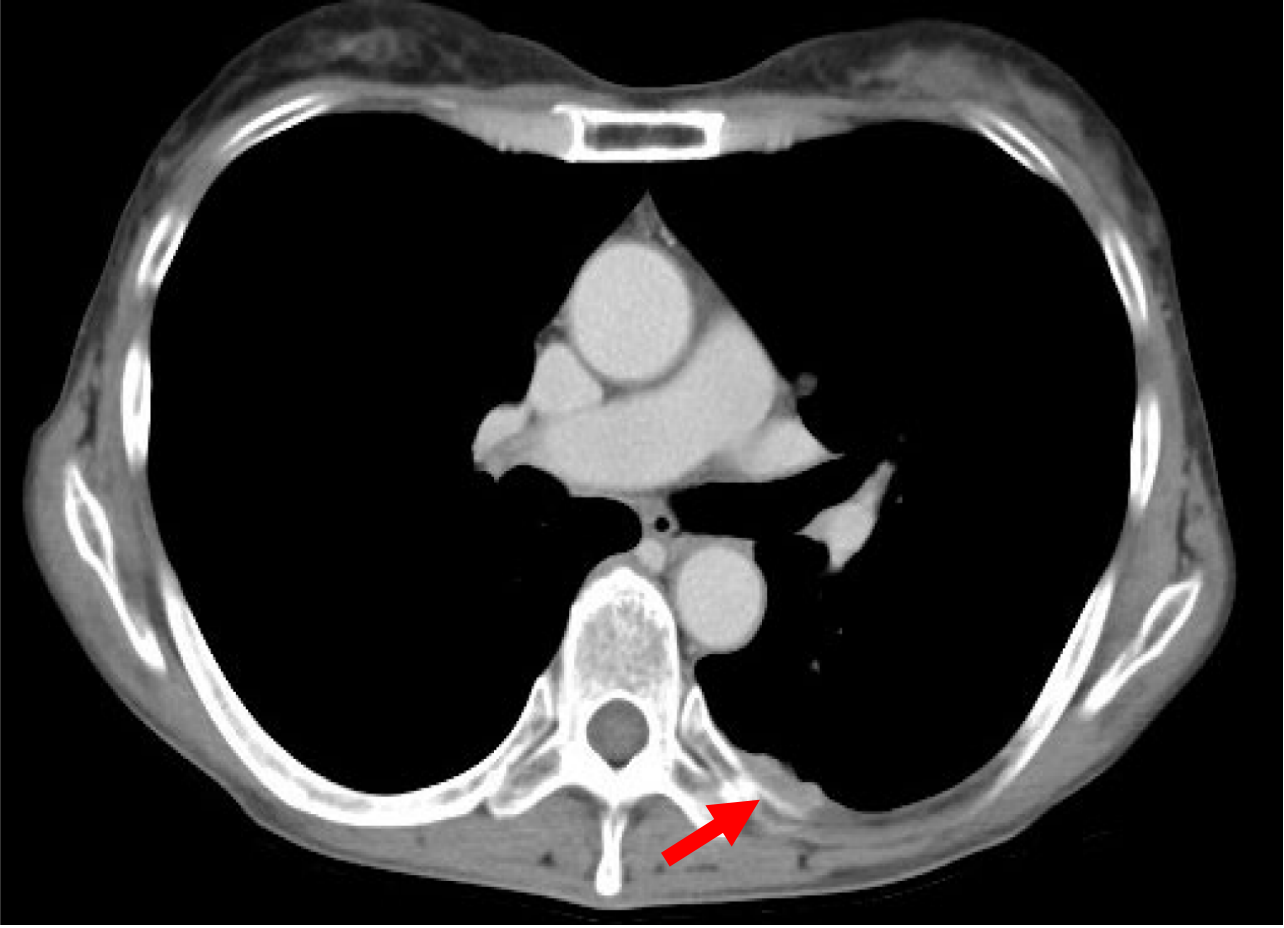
CT tomography shows a pleural nodule (red arrow).
